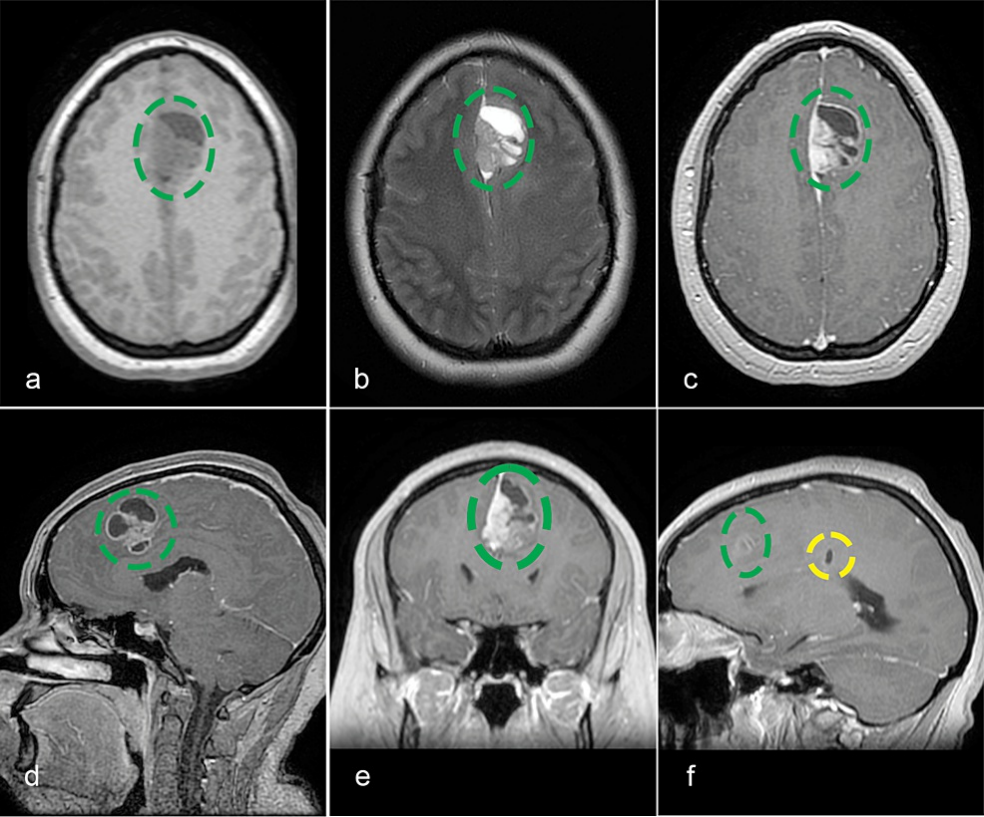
Enhanced MRI shows a left frontal parafalcine cerebral tumor (green circle). a: axial T1; b: axial T2; c: axial contrast-enhanced T1; d: sagittal contrast-enhanced T1; e: coronal contrast-enhanced T1; f: sagittal contrast-enhanced T1 depicting a small cystic lesion (yellow circle) in the left parietal lobe next to the lateral ventricle
